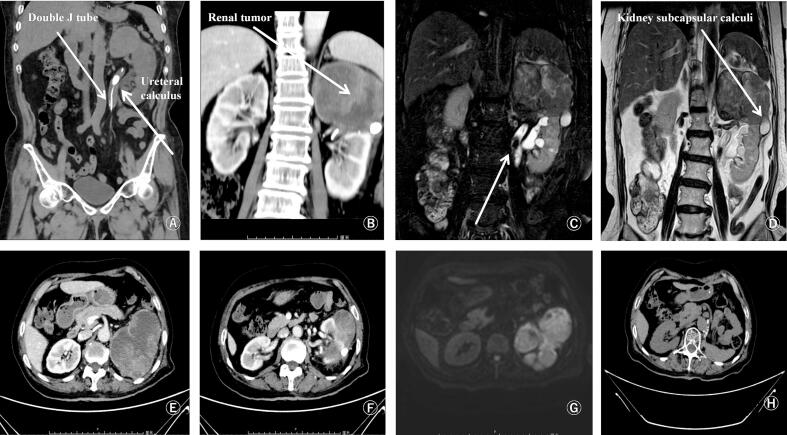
Preoperative and 6 month postoperative images. A: Preoperative CT scan showed left ureteral stent placement and left renal pelvis and ureter calculi. B: Preoperative enhanced CT showed rupture of the left upper kidney and renal subcapsular calculi. C, D, G: preoperative magnetic resonance imaging showed left duplex pelvis and ureter, upper ureteral calculi, renal subepithelial calculi, upper kidney and upper ureter occupying lesions; E, F: abnormal enhancement of the upper left kidney and ureter, possible mass; H: No abnormal CT scan was found 6 months after surgery.
